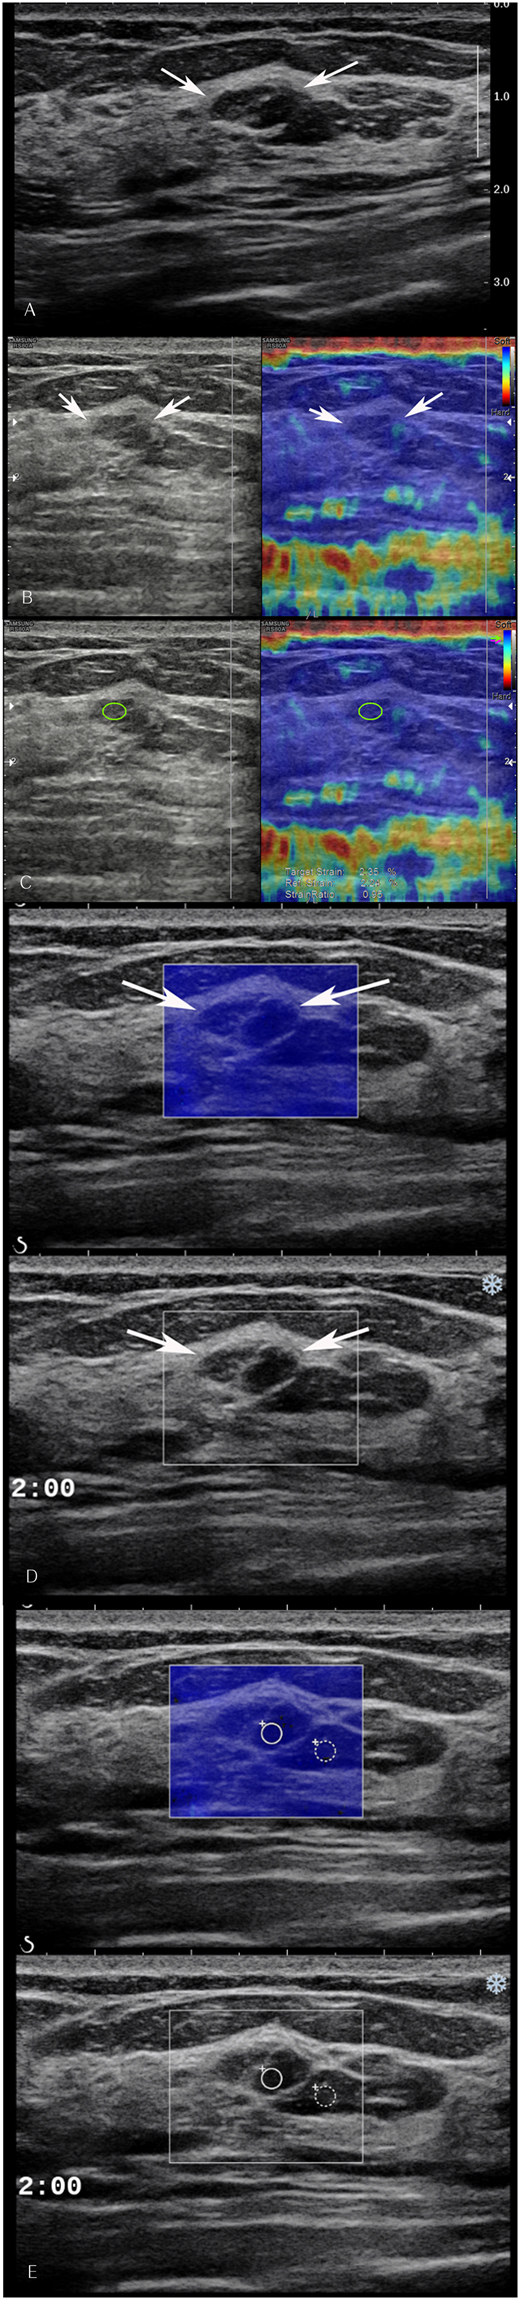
An abnormality detected via screening mammography in a 51-year-old woman. (A) Grayscale ultrasound shows an indistinct oval heterogeneous echoic mass (arrows) in the 2 o’clock area of the left breast which was categorized as a category 4a tumour with low suspicion of malignancy. (B) By strain elastography, the mass was graded with a visual score of 1 according to the Tsukuba system (arrows). (C) Strain ratio was 0.9. (D) By shear wave elastography, the mass was graded with a visual score of 1. (E) Elasticity ratio was 1.4. Ultrasound core biopsy confirmed the tumour to be a fibroadenoma.
